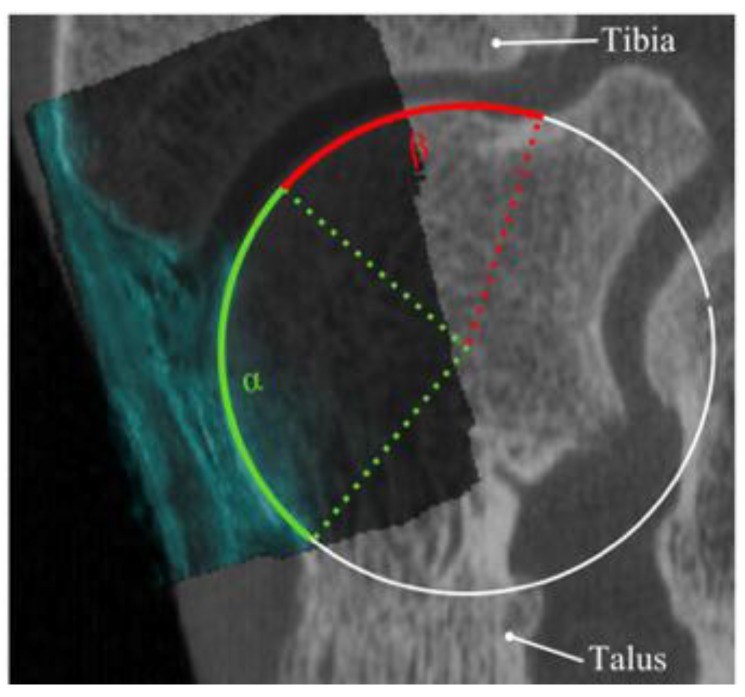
Sagittal view of one of the US volumes registered to the CT scan. The visible cartilage and cartilage covered by the tibia are represented, respectively, with *α* and *β.* The percentage of the visible cartilage was defined as the ratio of *α* to *α + β* (i.e., the total cartilage surface).
